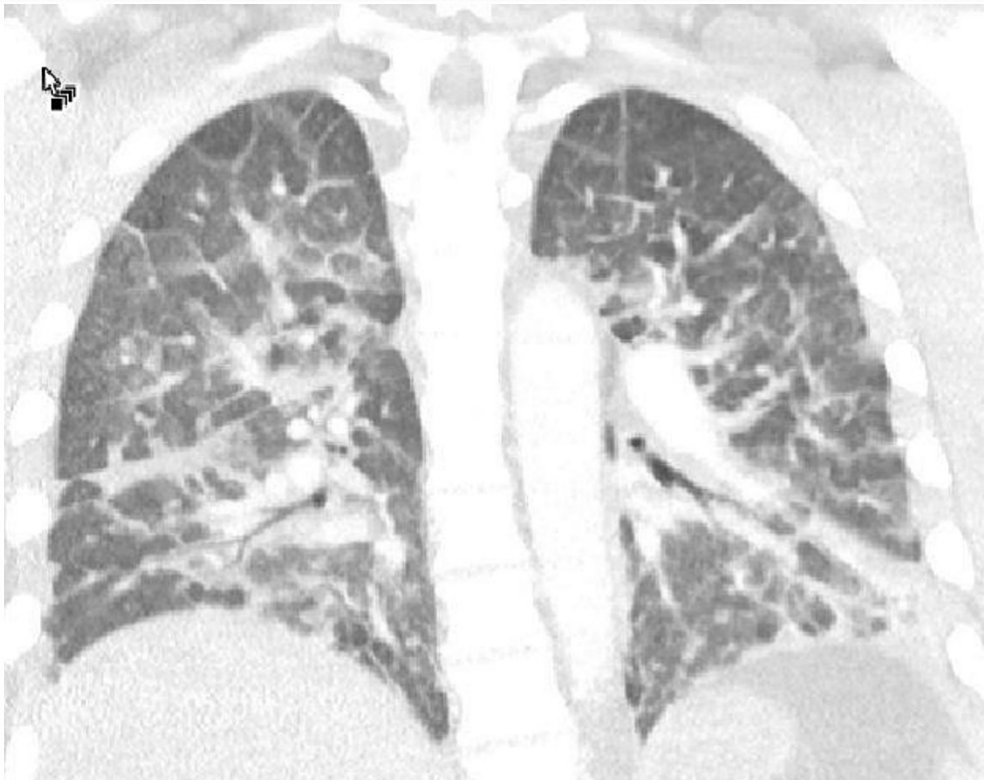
Initial CT angiography chest demonstrating multifocal lung infiltrates.
